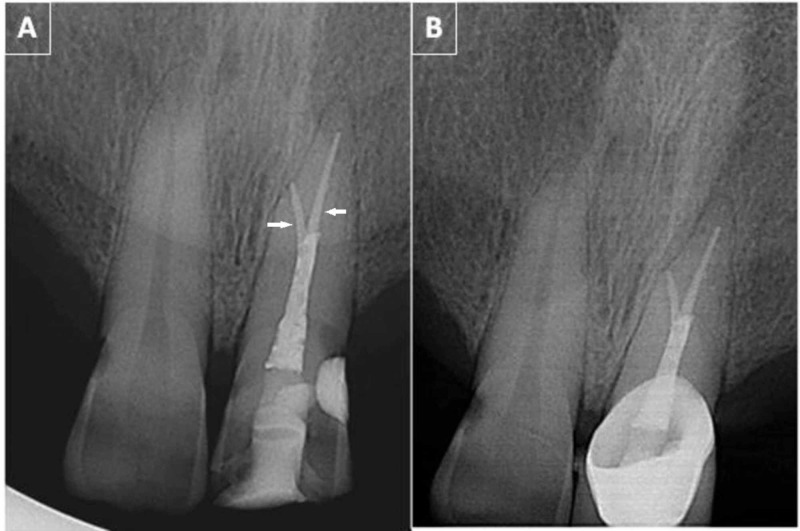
(A) Immediate postoperative radiograph of tooth number 21 showing well-obturated apical bifurcation (marked with arrows). (B) Twelve-month follow-up radiograph.
